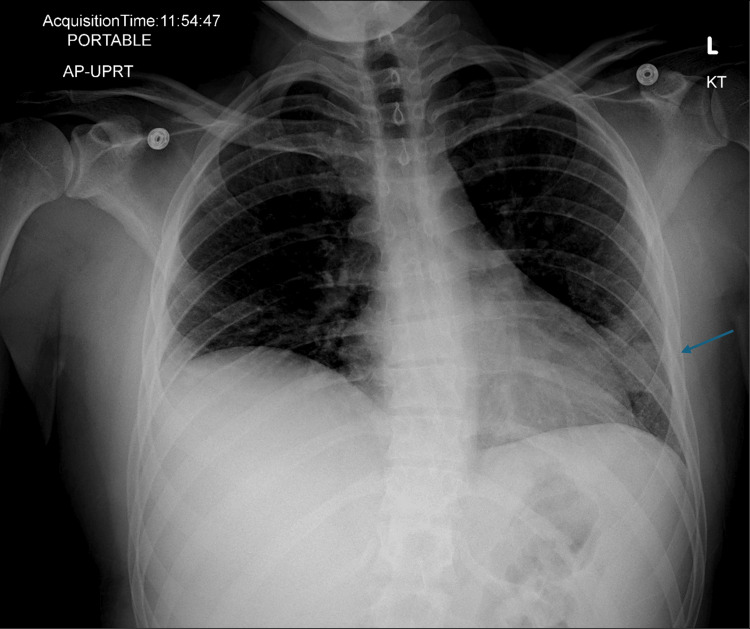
Chest radiograph showing elevated right hemidiaphragm and left lung infiltrate (arrow)
